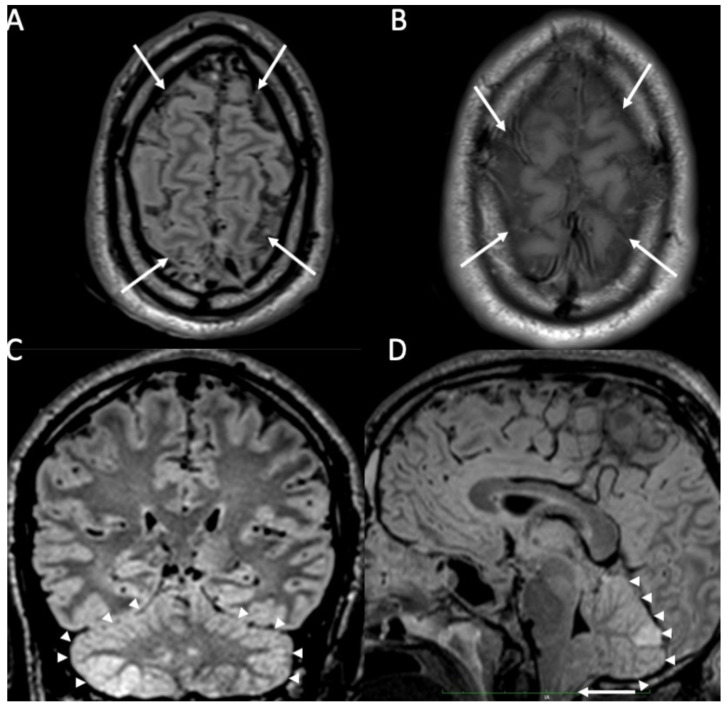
Axial FLAIR (A), T1 (B), coronal (C), and sagittal FLAIR (D) show diffuse hyperintensity on FLAIR and T1 of the subarachnoid spaces mainly in the cerebral convexity (arrows in A,B) in a patient with Streptococcus Pneumoniae meningitis. Note also concomitant cerebellitis, which appears as diffuse cortical hyperintensity on FLAIR of the cerebellum (arrowheads in C,D), with herniation of the cerebellar tonsils (arrow in D).
